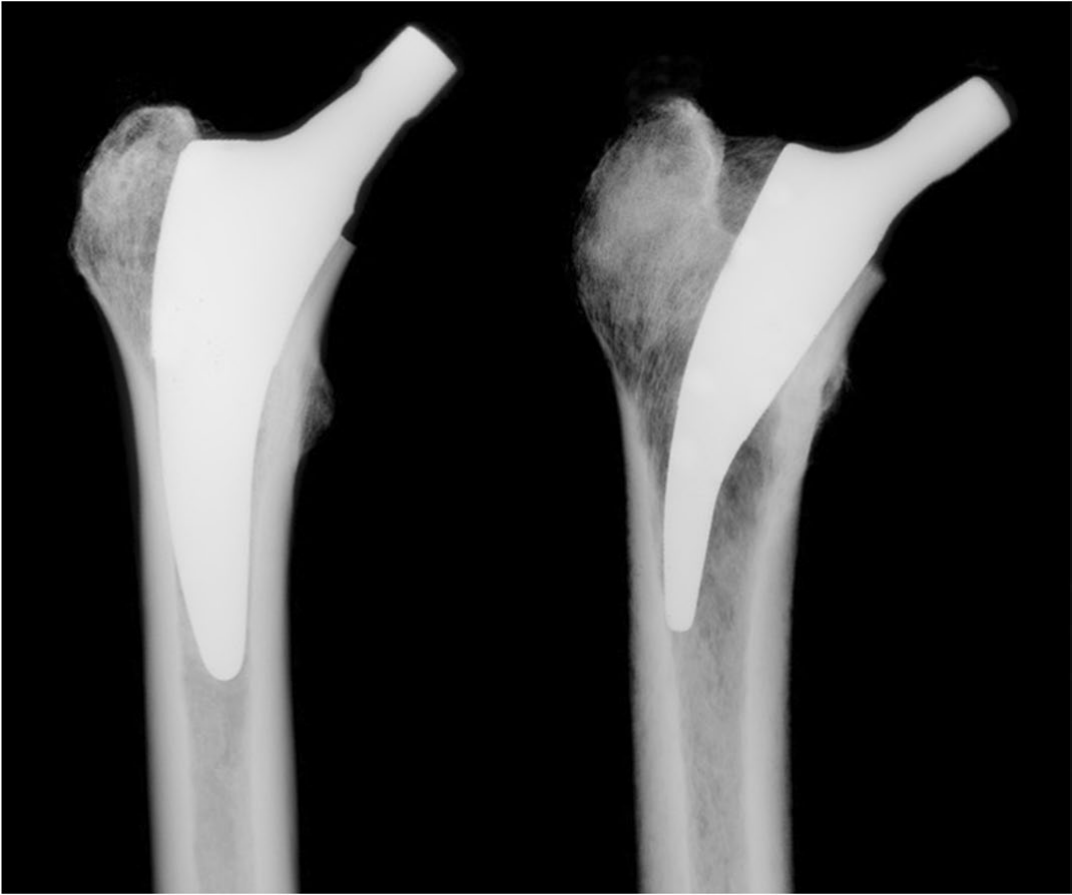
Radiographs of the Fitmore (left) and the Metha stem (right)
